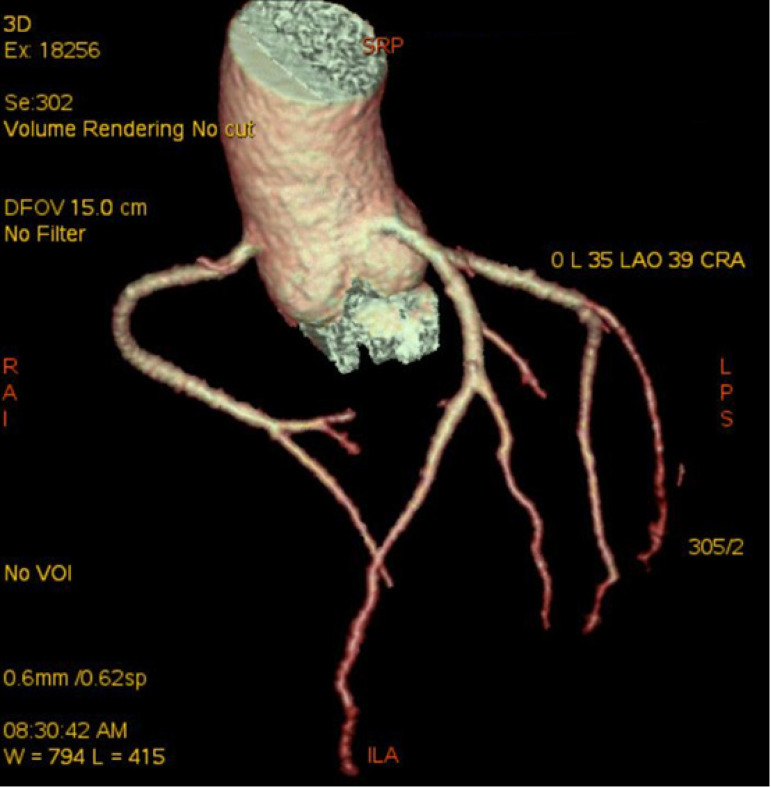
At 3 months postoperatively, the closure of the fistulae was confirmed by 128-slice computed tomography
